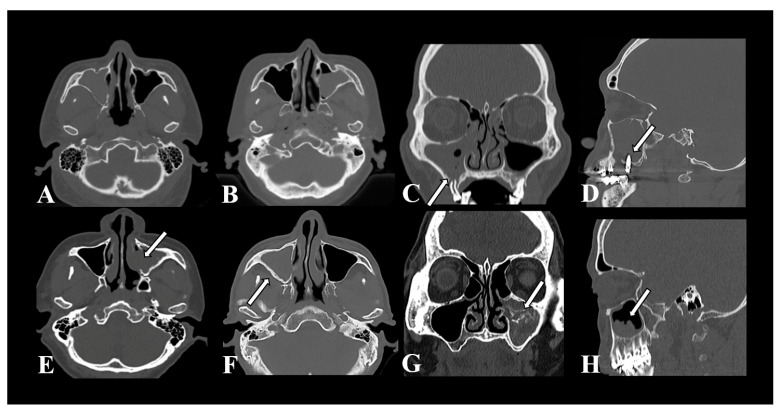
Computed tomographic imaging features of unilateral maxillary sinus lesions. (A) total opacification, (B) partial opacification, (C) periodontal bone loss, (D) penetrating dental implant, (E) erosion of the medial sinus wall, (F) sclerosis of the lateral sinus wall, (G) intralesional hyperdensity, and (H) irregular surface of the material.
